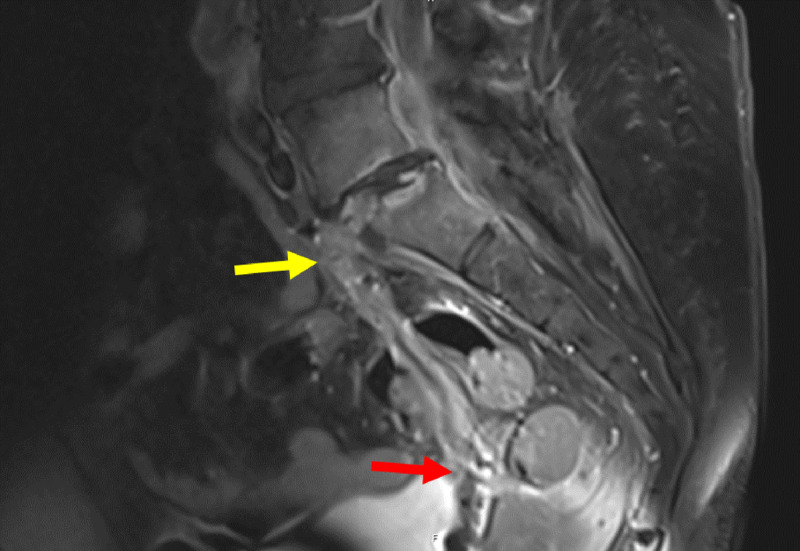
Sagittal contrast MRI in T1 weighting: Pre-operative MRI showing an epidural collection behind the vertebral body of L5 and within the L5-S1 disk (yellow arrow), fistulising into the vagina (red arrow).
